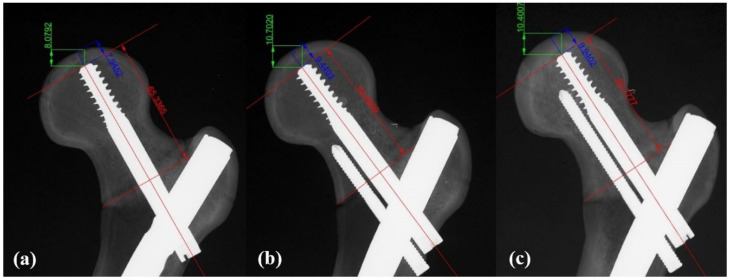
Anteroposterior radiographs showing the novel cephalomedullary (CM) nailing with three different fixation methods: (a) lag screw (100 mm) only, (b) lag screw with a short inferior anti-rotation screw (75 mm), and (c) lag screw with a long inferior anti-rotation screw (95 mm); the short anti-rotation screw does not pass through the femoral neck region and ends at the narrowest part of the femoral neck. Sliding distance between red arrows, cranial migration between green arrows, axial migration between blue arrows.
